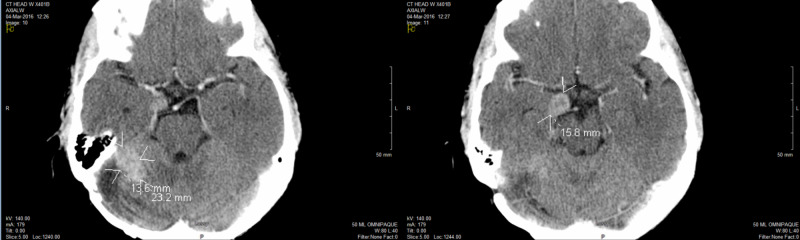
Post-craniotomy brain CT showing recurrent mbMets before the second WBI in 2016 mbMets, multiple brain metastases; WBI, whole brain irradiation
